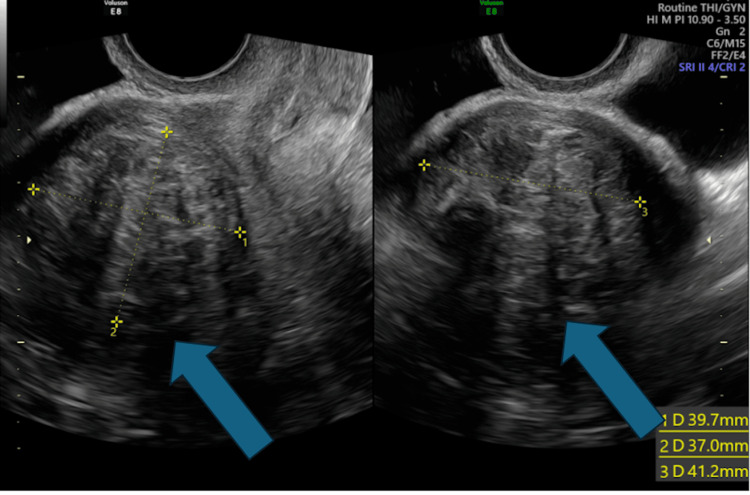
Transvaginal ultrasound: An anterior intramural fibroid is visualised, measuring 39.7 x 37 x 41.2 mm, in different axes as shown by the blue arrows.
